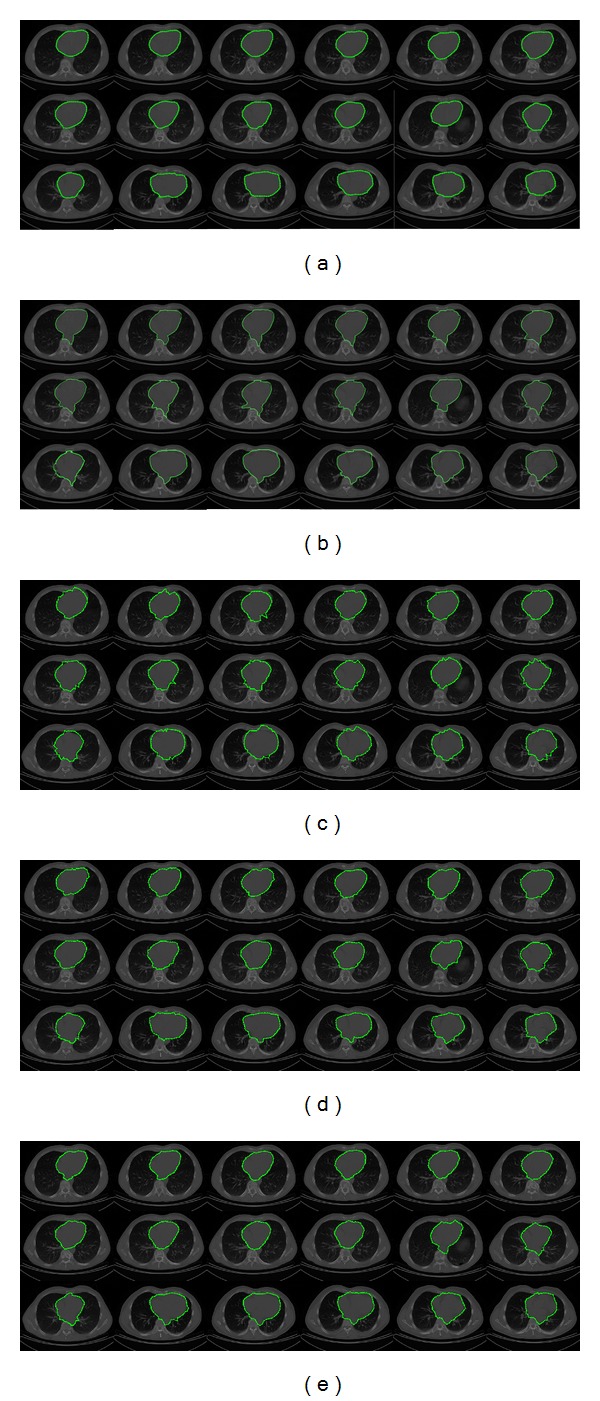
CT images (human heart segmentation): (a) manual delineation by experts, (b) results of Graph Cut method, (c) results of traditional ACM, (d) results of interactive Tseng method, and (e) results of proposed implementation.
